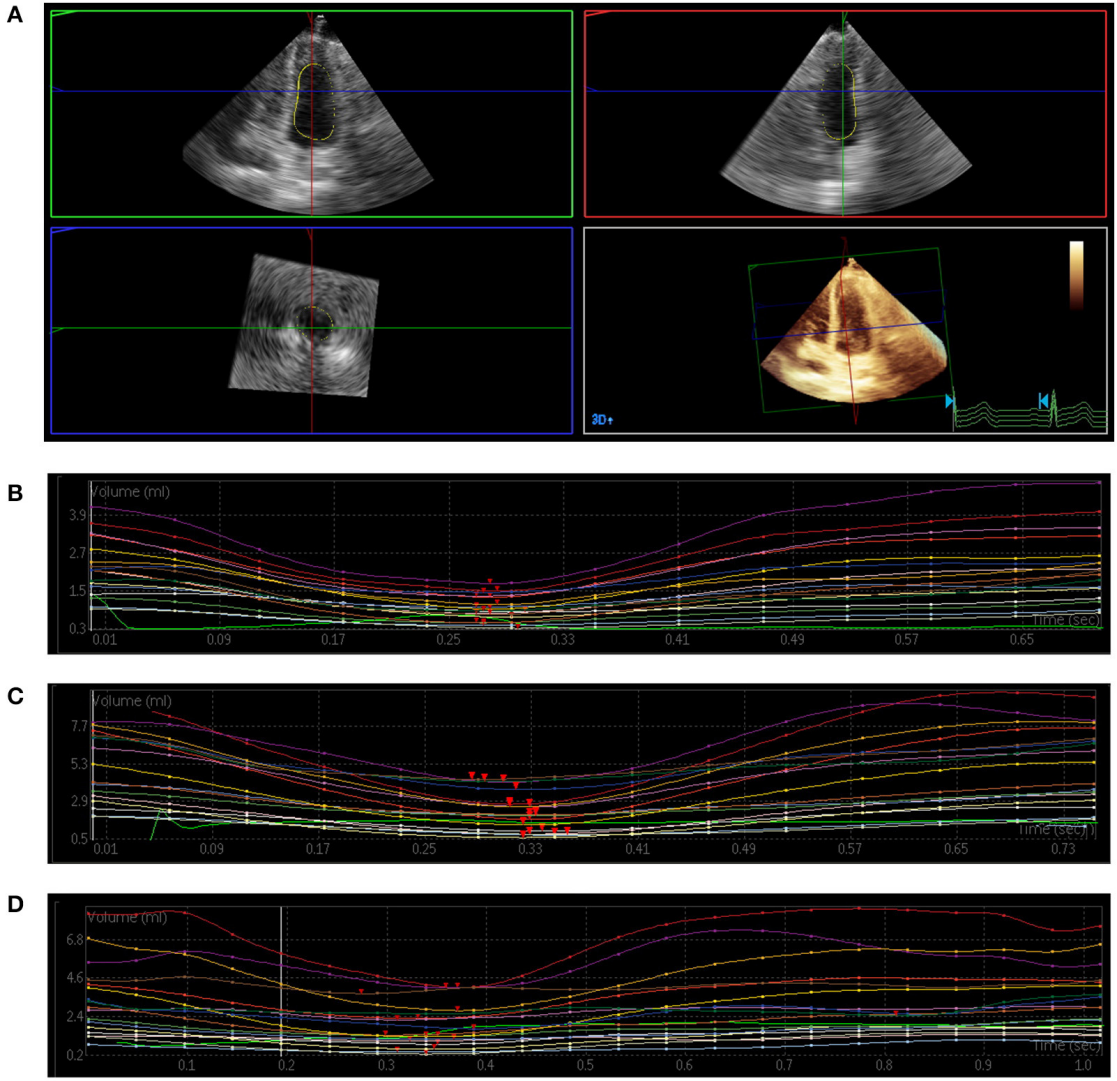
Three-dimensional echocardiography assessment of left ventricular (LV) mechanical dyssynchrony. (A) Full-volume acquisition. (B) Patient with normal intraventricular synchrony. There is a regular time–volume curves, and the time of reaching the trough is consistent. (C) Patient with slightly intraventricular dyssynchrony. The time–volume curves is regular but not consistent. (D) Patient with severe intraventricular dyssynchrony. There is a disarray of the time–volume curves signifying LV dyssynchrony.
